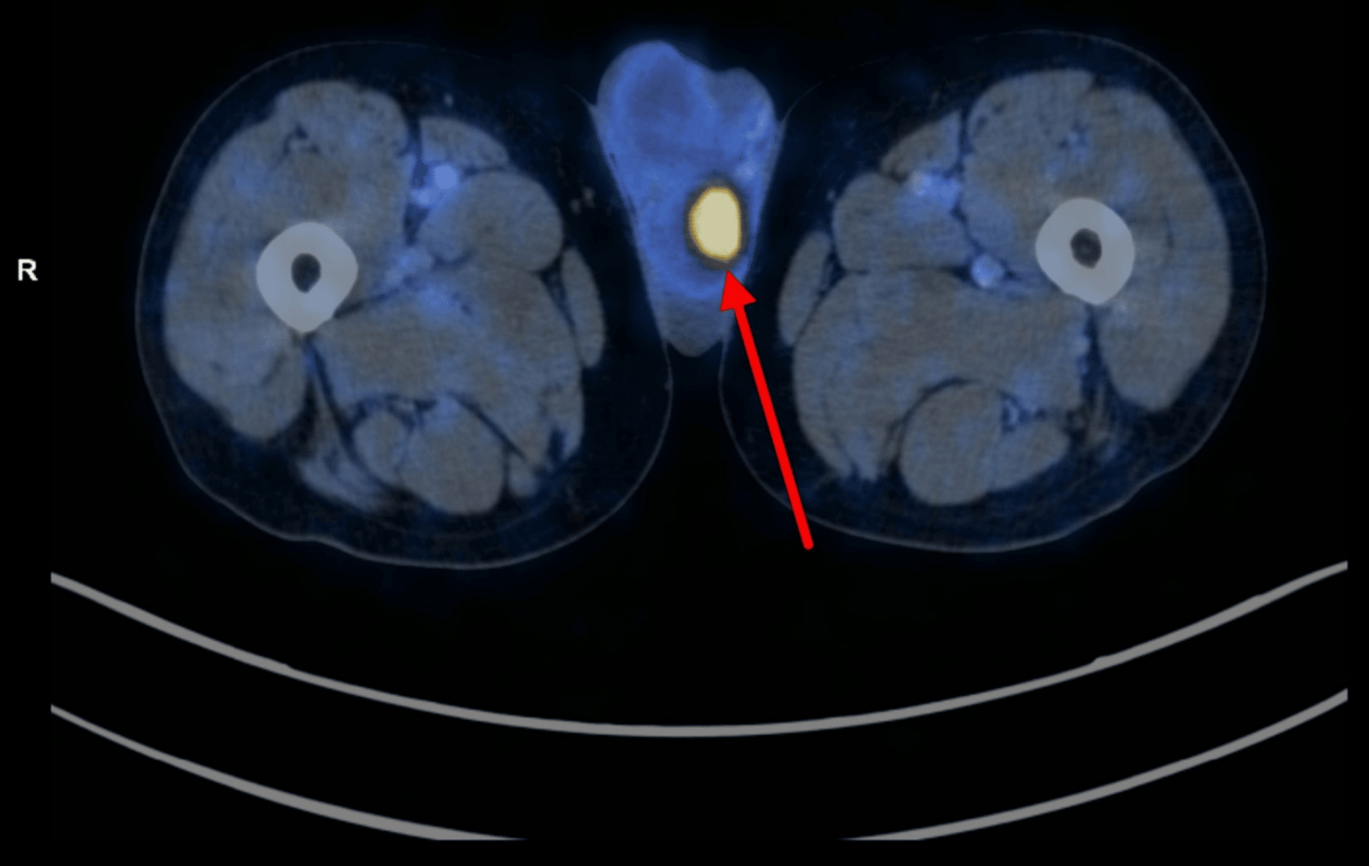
Fused PET-CT axial slice showing a focally intense PSMA-avid lesion in the left testicular region. PET, positron emission tomography; PSMA, prostate-specific membrane antigen; CT, computed tomography
